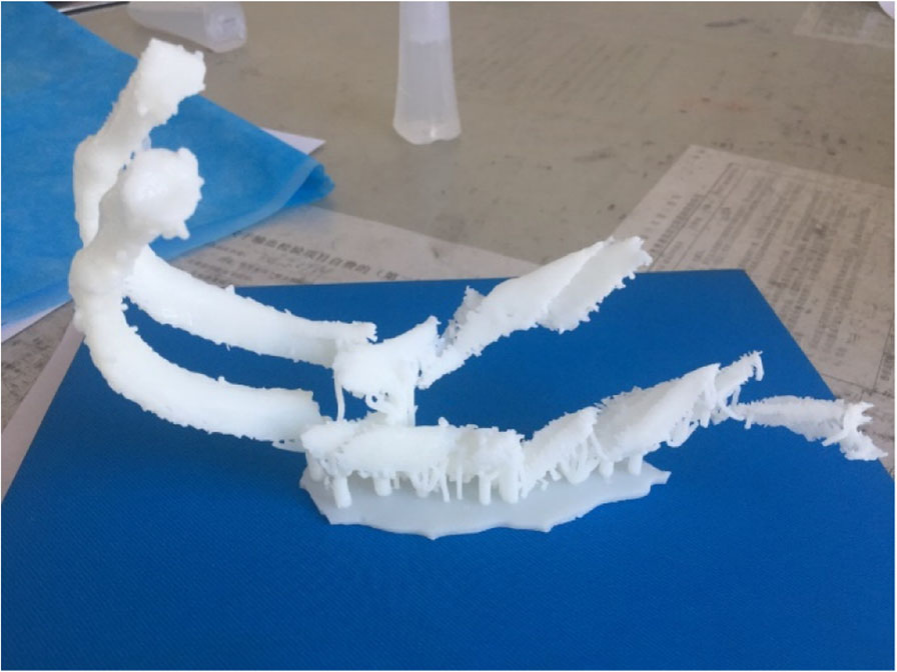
Preoperative Reconstruction of the 3D model from CT thin-layer scan results prepared by 3D printing technique. Preoperative 3D reconstruction of the 3rd and 4th rib images; 3, 4 rib model prepared by 3D printing technique.
